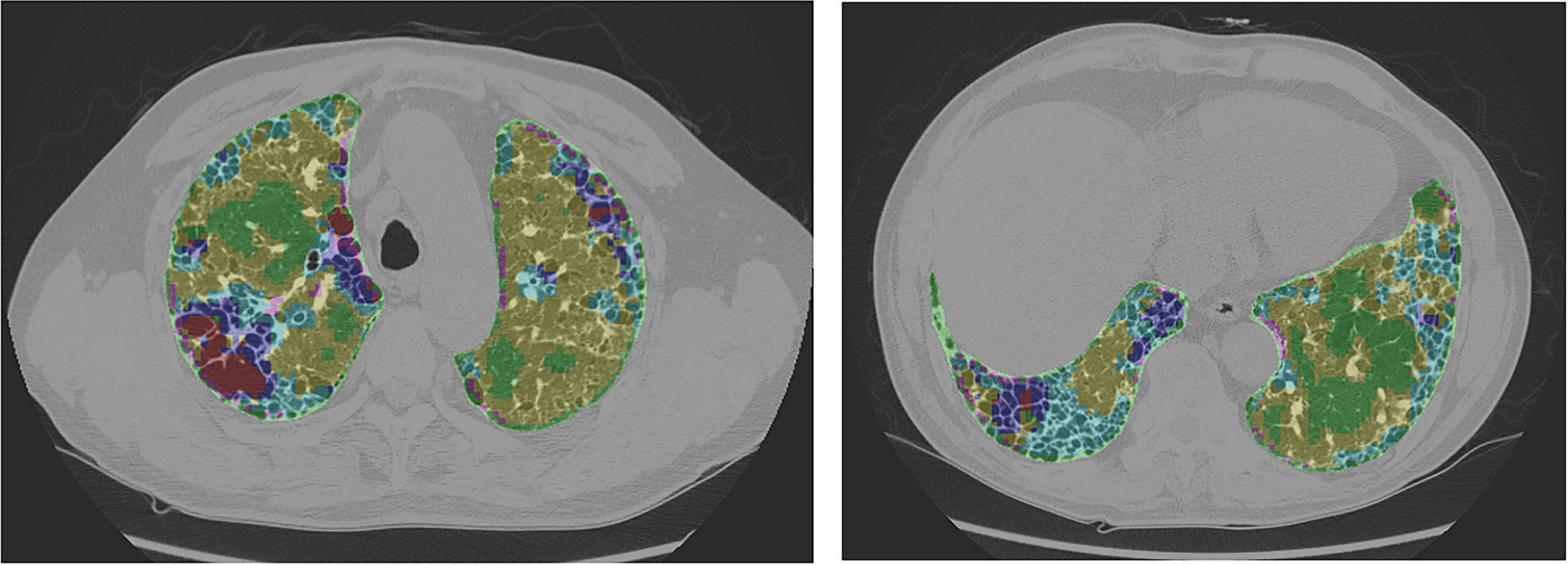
Texture-based automated quantification was performed on volumetric thin-section CT in patients with IPF and shows the extent of regional patterns with different colors. Green = normal, yellow = ground-glass opacity, cyan = reticulation, red = emphysema, pink = consolidation, blue honeycombing. IPF = idiopathic pulmonary fibrosis; CT = computed tomography.
